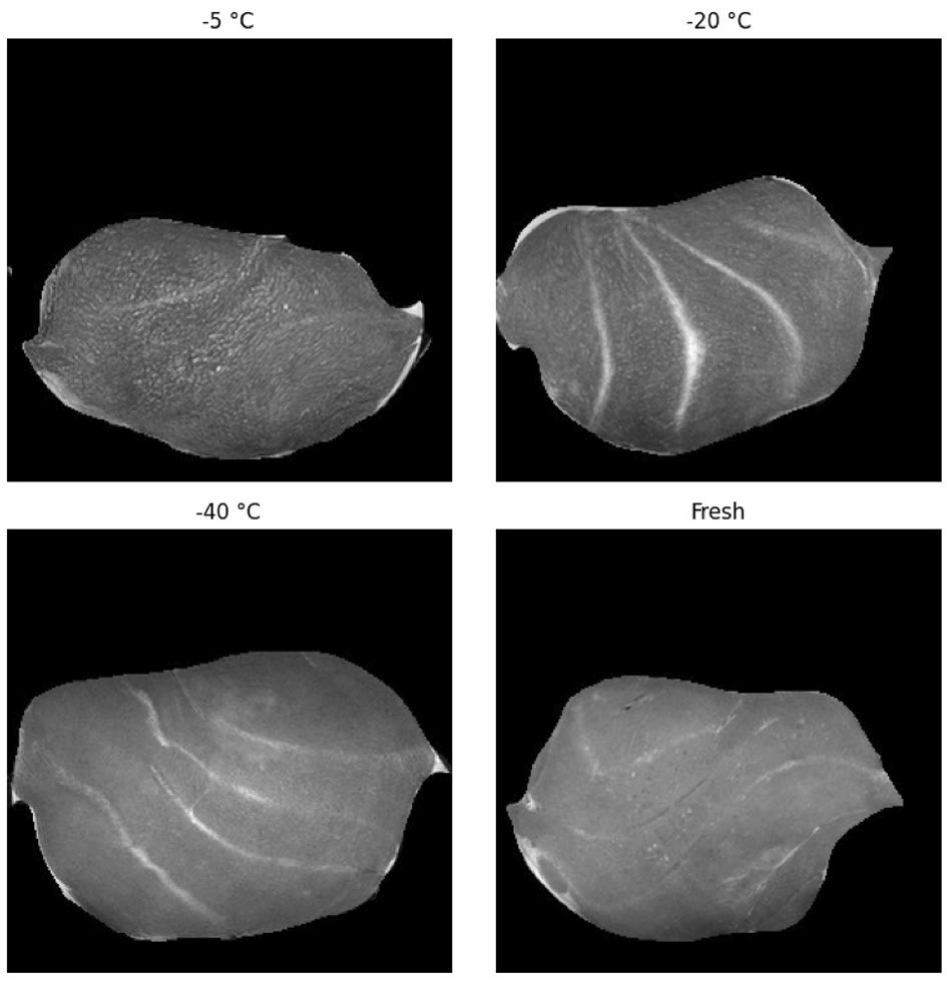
Examples of $$T_{2}$$-weighted MRI image slices for samples that underwent a − 5 °C, − 20 °C, or − 40 °C freezing and thawing process, as well as a $$T_{2}$$-weighted MRI image slice in the fresh state (i.e., obtained before freezing and thawing).
